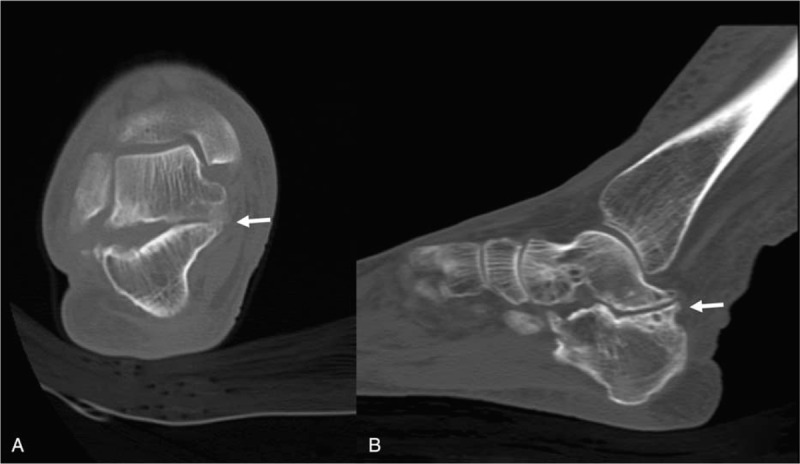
Computed tomography (CT) scan of the right ankle. Oblique coronal CT (A) and sagittal CT (B) showed irregular medial posterior facet, partial coalition, narrowing, and subcortical cyst formation at the posterior subtalar joint (arrow).
